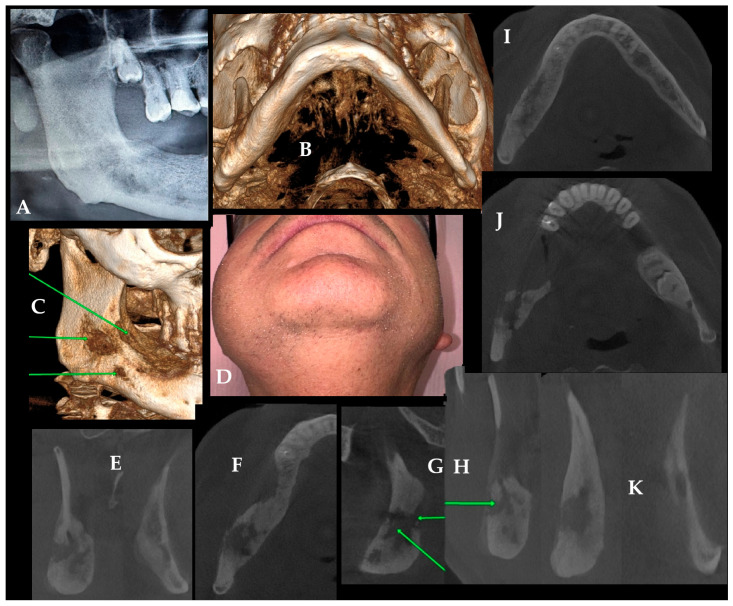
Long-standing chronic osteomyelitis with marked mandibular remodeling and clinicoradiological progression. Quite an important remark should address that chronic osteomyelitis (COM) may persist for many years and, in rare cases, can present clinical and radiological features that overlap with aggressive bone lesions, including osteosarcoma. Although such an association is extremely uncommon, this highlights the importance of close follow-up in patients with longstanding COM, particularly when progression or atypical findings are observed on clinical or radiological examination. In this case, wisdom teeth 48 with a cyst formation were removed more than ten years ago. Significant local bone inflammation was treated for years, without any sufficient bone revision, biopsy, or approach towards the bone asymmetry, swelling, facial pain, and presence of COM. It is quite important to understand that despite significant patient facial swelling (D), routine DPR (A) provided only limited radiological information and underestimated the extent of disease. In this case, DPR raised only a general suspicion of mandibular pathology, whereas CBCT clearly demonstrated cortical destruction, bone remodeling, periosteal reaction, and the true three-dimensional extent of the lesion. These additional CBCT findings were clinically important because they supported the decision for surgical revision, biopsy, and microbiological sampling rather than continued conservative management alone. In a routinely performed CBCT, loss of both cortices, mandibular angle asymmetry, and swelling with bone remodeling, suggested progression of COM over the years (B,C). Presented COM is quite similar to Garre-like osteomyelitis with a very long time of symptom occurrence (green arrows indicate irregular bone structure loss). Chronic pain, extensive swelling and some worrisome clinical symptoms forced the patient to seek help in our ward. Mandibular asymmetry with bone loss and remodeling (E–J) was present. A comparison of right-to-left mandibular angle (K) shows the scope of bone changes with remodeling, loss of its trabecular lining, and periosteal elevation. The patient was scheduled for a bone revision under general anesthesia. The procedure consisted of bone ostectomy, the Obwegeser-Trauner approach, bone saucerization, ostectomy, and debridement. The bone was shapeless, more osteoclerotic, with local softening, and presented a COM for a long period of time. Surgery consisted of bone re-shaping, modeling, and providing a lot of bone samples to confirm the COM and exclude any OSA formation, as well as to establish microbiological flora. Additional antibiotic bone-sponges were applied with anticoagulant therapy for ten days. Patient remains free of unwanted bone inflammation and healed with good outcomes. The residual bone following excision remains under observation, and the patient has been informed about the potential need for a second revision surgery and additional bone decortication if necessary. The presented COM case emphasizes that adequate early bone revision, irrigation, and surgical debridement immediately after wisdom tooth and cyst removal may lead to more favorable outcomes in the early time-frame. Local bone infections originating from wound irritation or untreated alveolitis may lead to chronic osteomyelitis (COM) if not managed in time, as presented in Figure 4. Antibiotic therapy alone should not be considered as the only approach to any bone complications without its surgical revision, debridement, ostectomy, and flap coverage.
